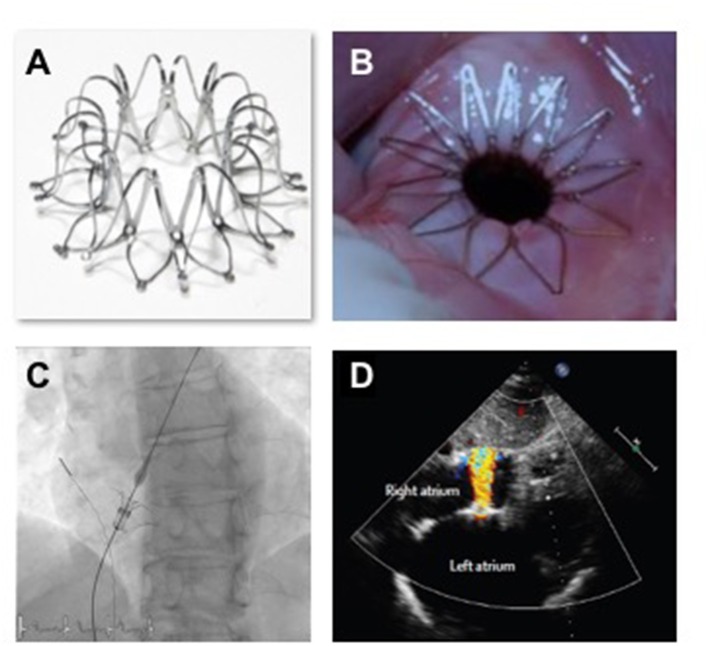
Images (A,B) show the IASD; (C) show the fluoroscopic image of IASD delivery; (D) echocardiographic image showing left to right blood flow.
